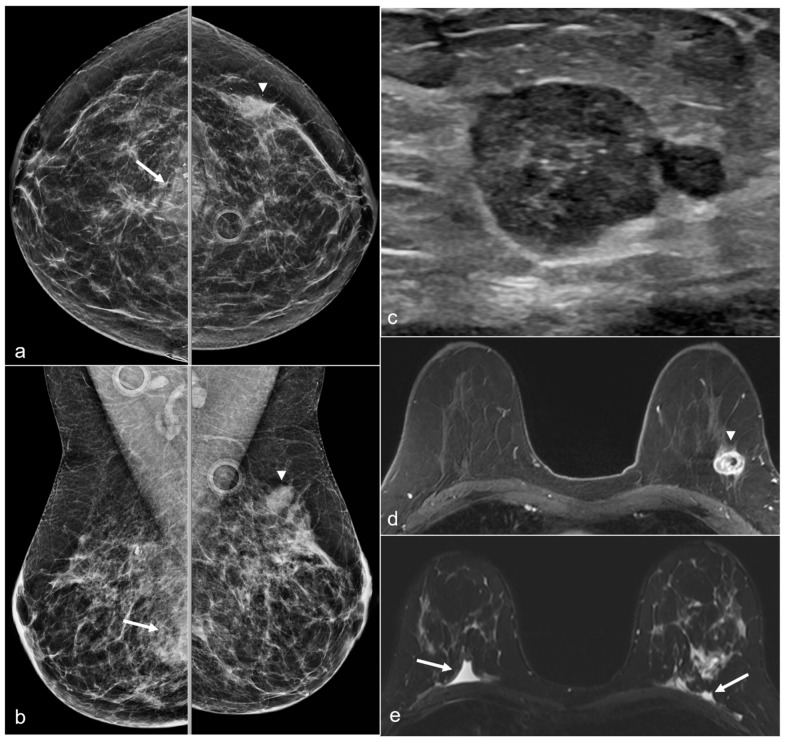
A 50-year-old woman with a history of subglandular silicone implants, status post BES. Screening mammograms with bilateral CC (a) and MLO views (b) demonstrate a mass in the left breast upper outer quadrant (arrowhead). BES changes are more prominent in the right breast with focal asymmetries in the prepectoral region (arrows) with dystrophic calcifications. Subsequent ultrasound examination of the left breast in the transverse plane (c) with ultrasound-guided biopsy revealed triple-negative invasive ductal carcinoma. T1-weighted post-contrast MRI (d) revealed a left breast enhancing mass (arrowhead) with a biopsy clip. There was also right greater than left prepectoral fluid (arrows) on the axial T2-weighted images (e).
